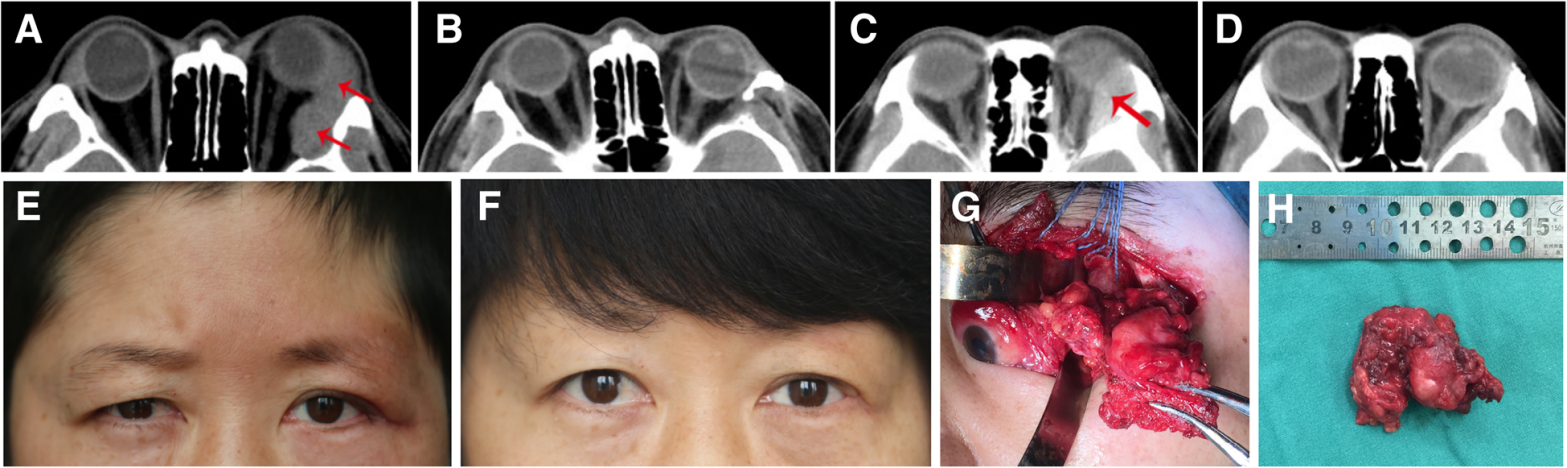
CT scan of Case 9, who had adenoid cystic carcinoma of the lacrimal gland, and axial computed tomographic scan after surgical resection (a, b). Photos and CT scan of Case 20, who had adenoid cystic carcinoma of the lacrimal gland, and axial computed tomographic scan after surgical resection (c, d, e, f). Intraoperative photograph for eye-sparing tumor excision (g). Excised tumor (h)
